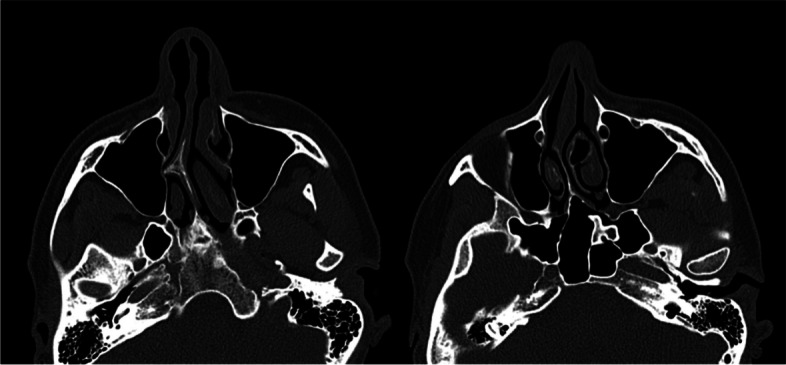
CT of the external nose and nasal cavity prior to CT-assisted precision rhinoplasty of patient B who initially appeared for rhinoplasty solely for aesthetic indications, depicting (a) large nasal spur, hypertrophic left lower and middle turbinates, (b) narrow internal nasal valves, high septal deviation, left concha bullosa, thick soft tissues
